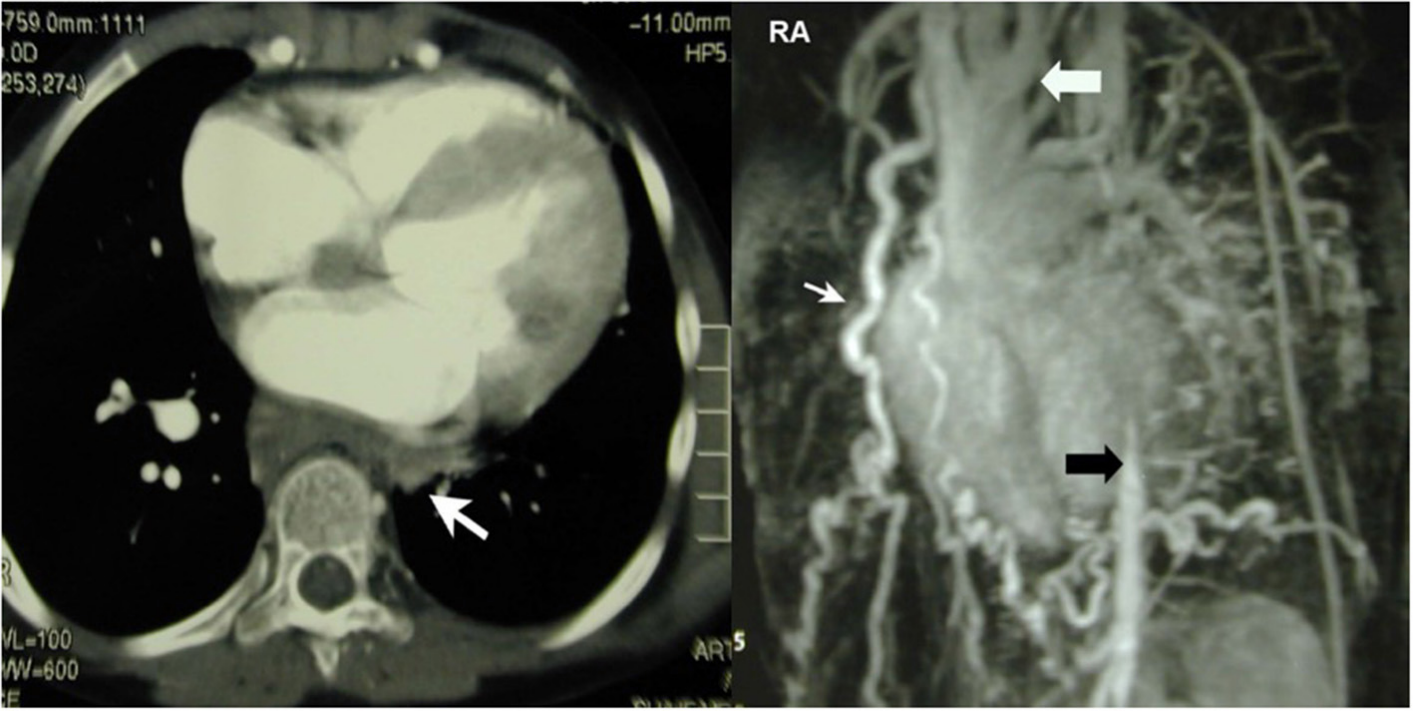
Left: CT-scan showing segmental agenesia (white arrow) of the descending aorta. Right: Huge network of collaterals. The missing segment lies between the distal aortic arch (white arrow) and the thoraco-abdominal junction (black arrow). Reproduced with permission from ICVTS 2003; 2:231–3.
